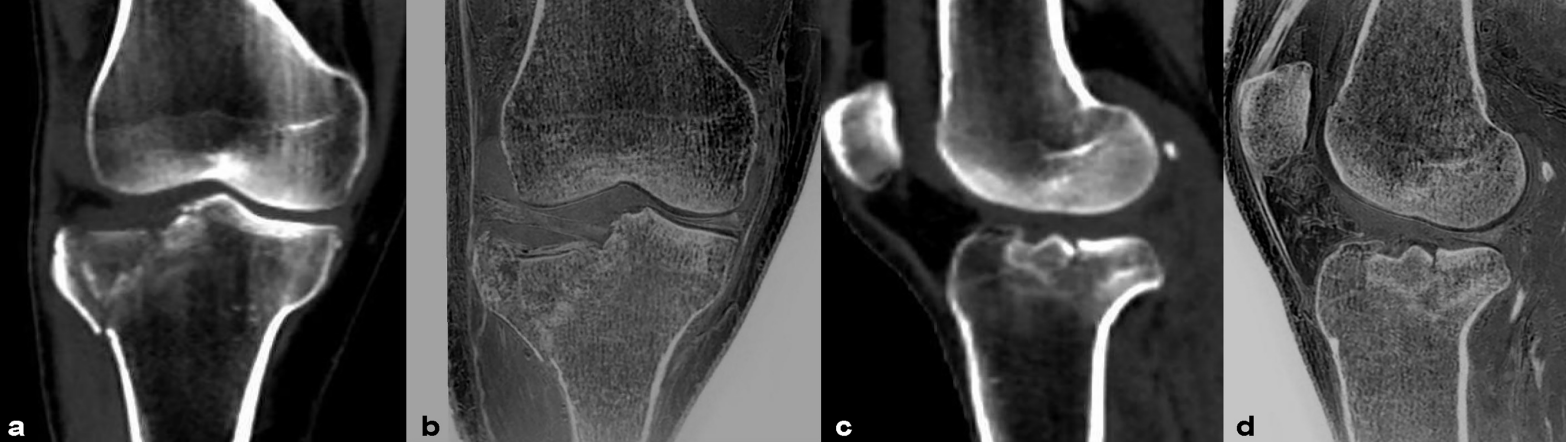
Images in a 45-year-old male with fracture of the right tibial plateau. (A, B) were coronal CT and FRACTURE images. (C, D) were sagittal CT and FRACTURE images. CT and FRACTURE images showed the same oblique fracture line and bone fragment displacement.
